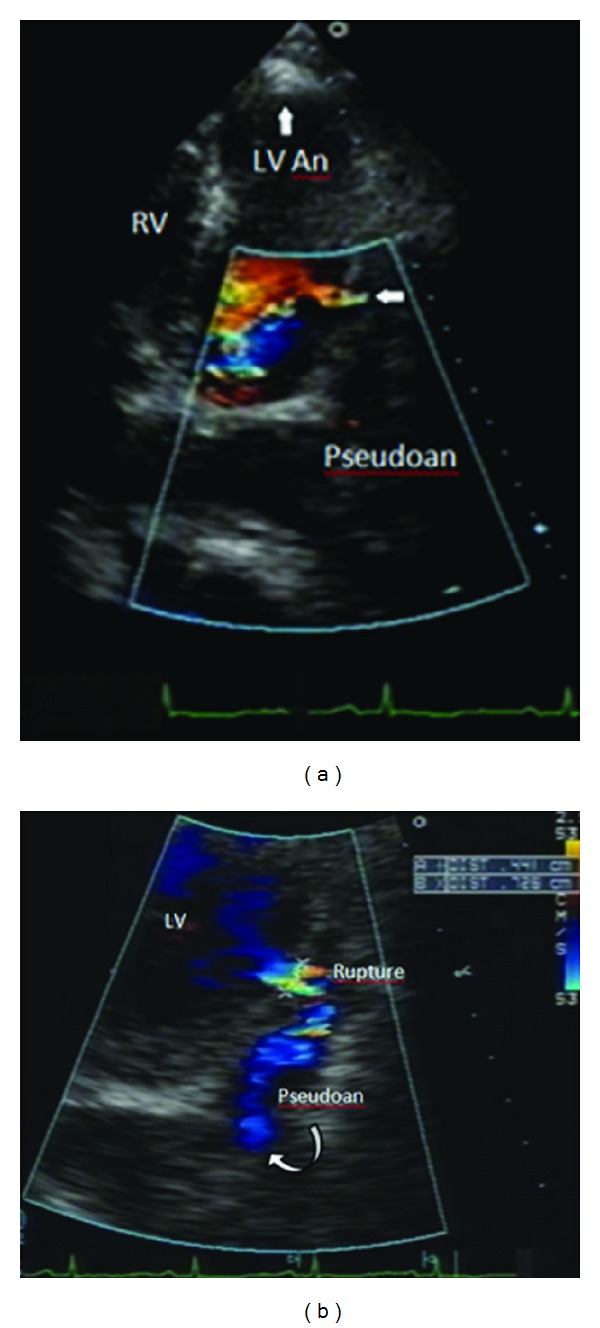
(a) Transthoracic two-dimensional and color Doppler echocardiogram in four-chamber view, showing the left ventricular apical aneurysm (LVAn), the site of rupture (arrow) and the pseudonaeurysm formation (curved arrow). (b) Zoom in lateral wall rupture with color Doppler.
